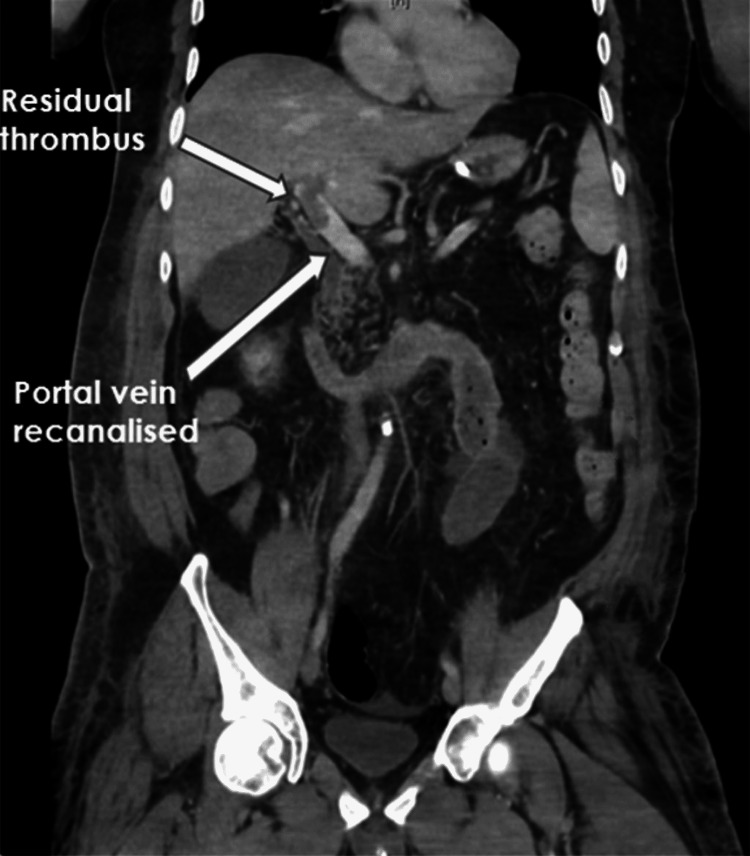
CT abdomen showing recanalisation of the portal vein.
